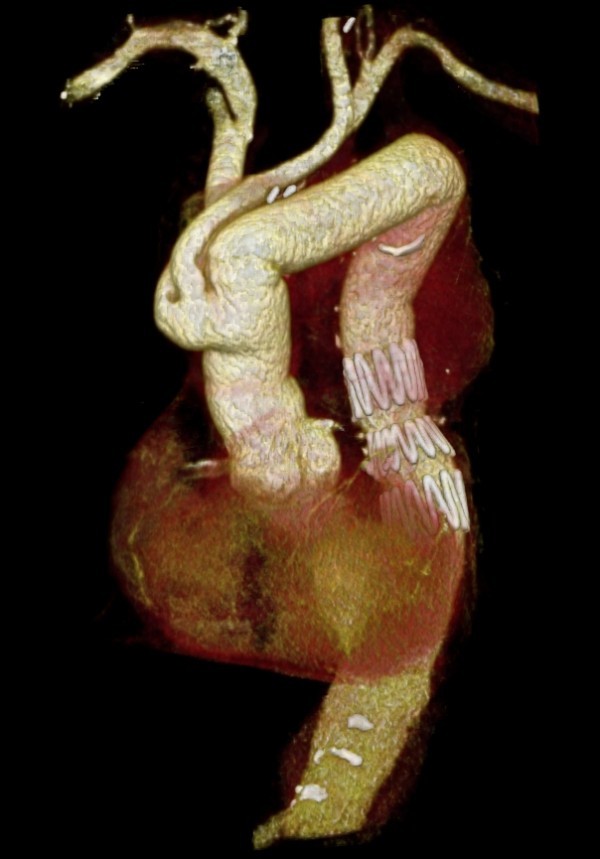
Three-dimensional computed tomographic reconstruction (2 years follow-up) demonstrating the occlusion of the by-pass between the two carotids.
